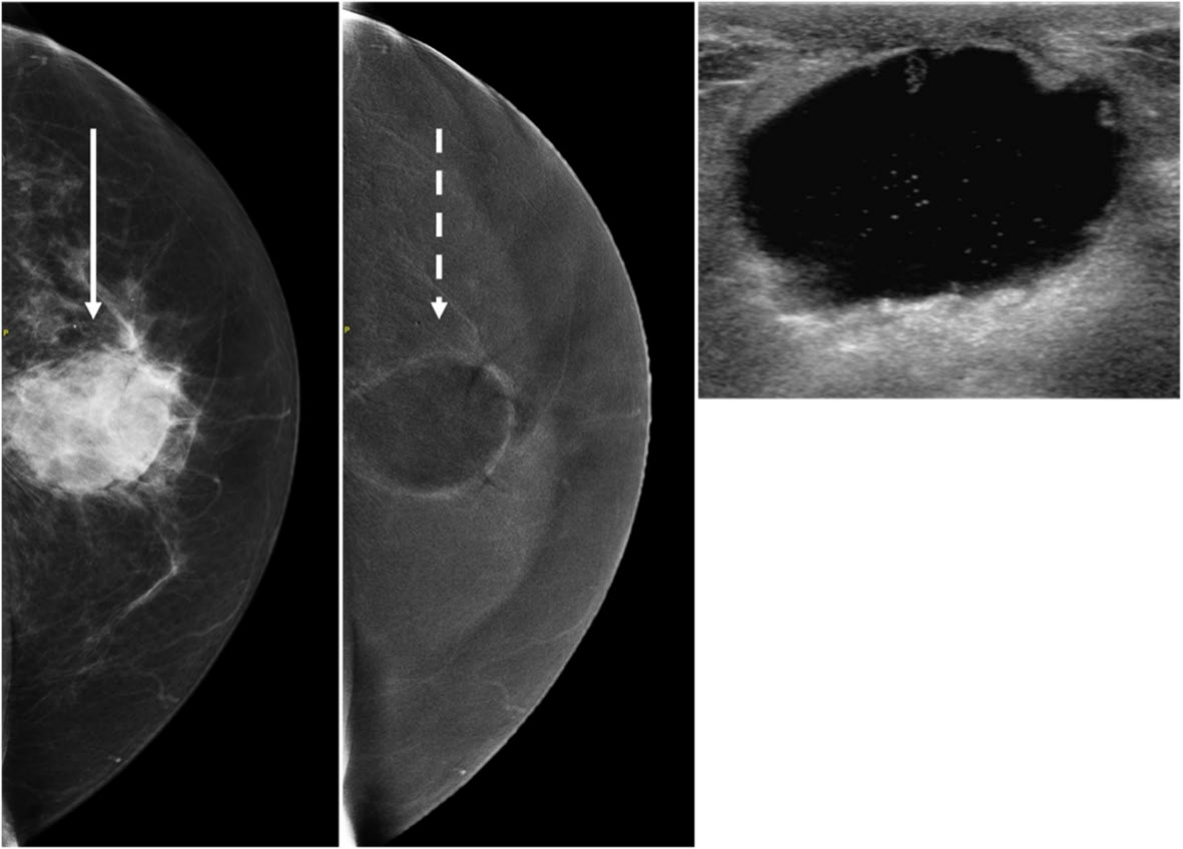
63-year-old woman with left breast palpable abnormality 7 months post excisional biopsy for atypia. CEM shows a dense round mass (solid arrow) on LE image in the central left breast with associated rim enhancement on RI (dash arrow). On subsequent ultrasound, a fluid collection with mildly thickened wall in the area of previous excision corresponds to the mammographic finding, supporting benign etiology of postoperative seroma
